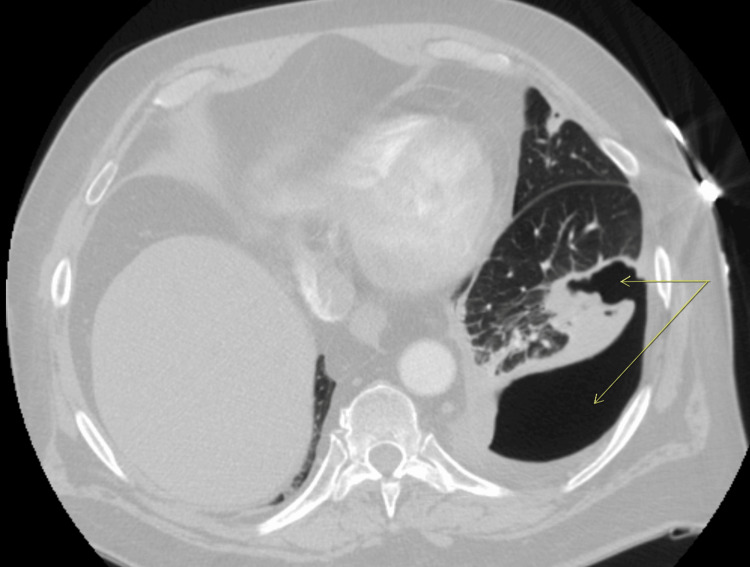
Axial CTA chest lung window showing a left lower lobe thick-walled cavity of 5.6 × 3.9 cm communicating with the adjacent pleura (arrows) CTA, computed tomography with angiography
